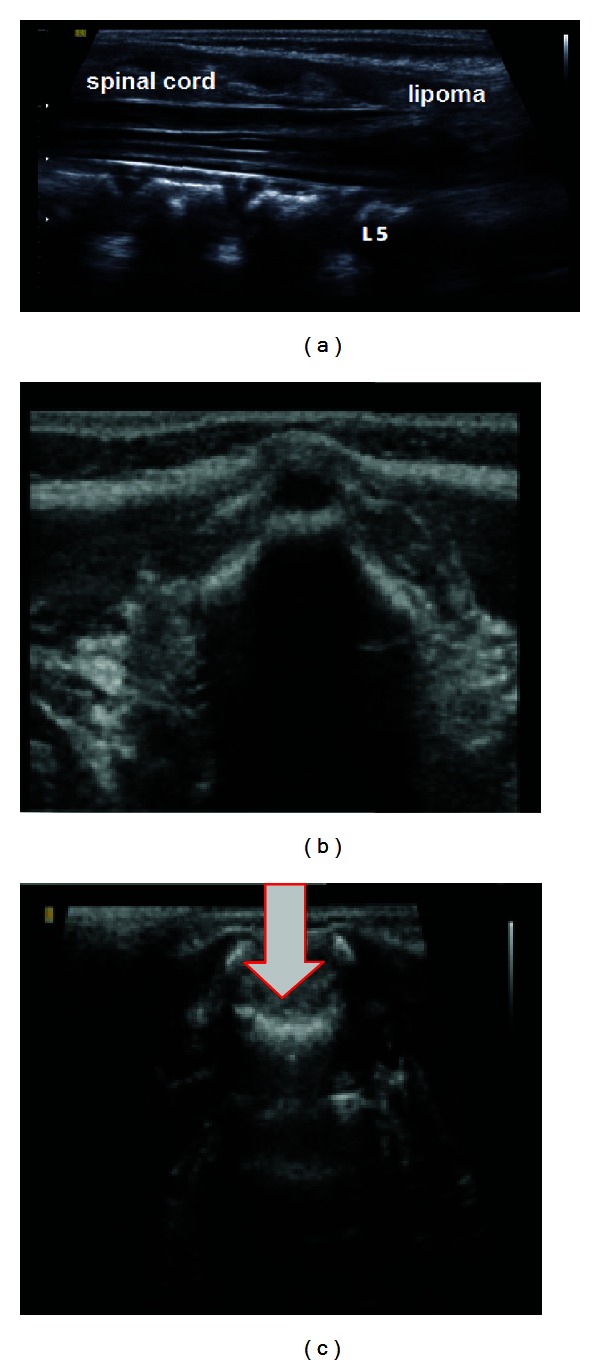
(a) Ultrasonography in the sagittal plane, showing a medullary cone extending as far as the sacral vertebrae, with a hypoechoic solid formation inside the canal (lipoma); (b) ultrasonography in the axial plane at the level of the upper lumbar spine (L1), showing a closed medullary canal; (c) ultrasonography in the axial plane at the level of the lower lumbar spine (L5), showing discontinuity of the posterior bone layers (white arrow).
